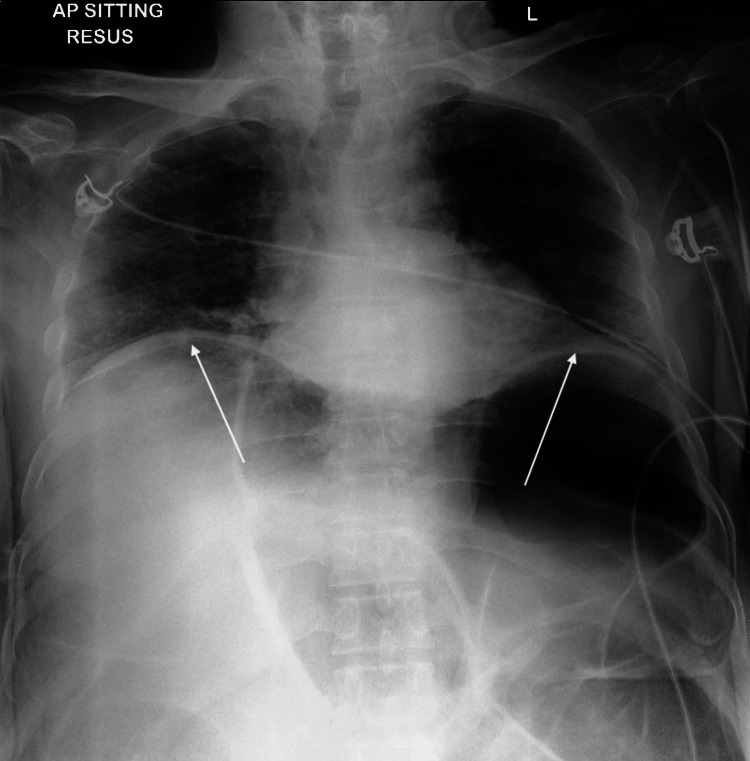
Decreased lung volume and cephalad displacement of diaphragm on chest X-ray
